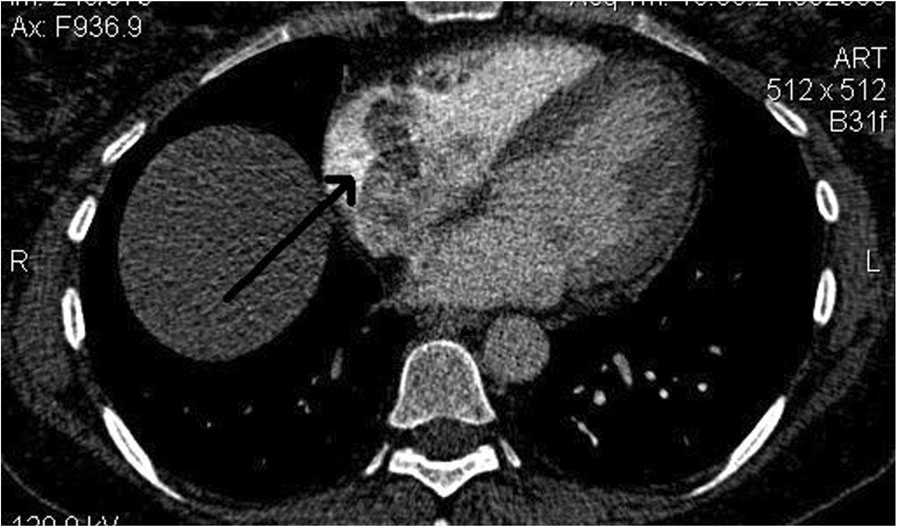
Computed tomographic angiography demonstrating the hypodense filling defect in the right atrium. The arrow is pointing to this filing defect
